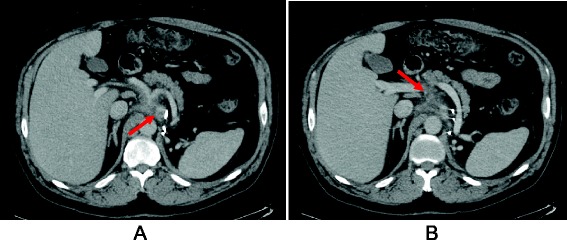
Baseline CT scan. Baseline CT scan showing the two selected target lesions as per RECIST 1.1 criteria: a left coeliac lymph node measuring 1.6 cm in the short axis (a) and a soft tissue mass next to the superior mesentery artery measuring 5 cm (b)
